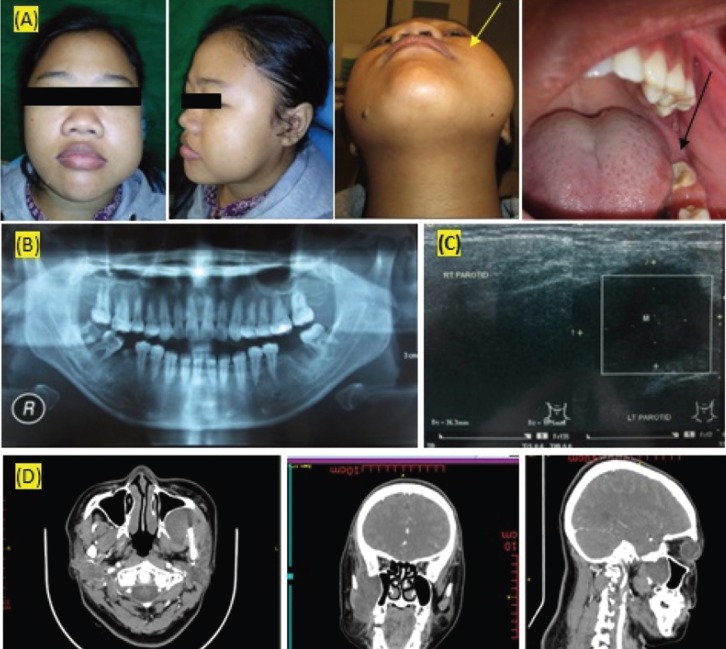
(A) Extra oral picture showed an asymmetry on the left cheek (black arrow) and (B) Orthopantomogram revealed no bone destruction of maxilla and mandible, (C) USG showed a hypoechoid solid mass on the left parotid area. (D) CT scan with contrast enhancement from coronal, axial, and sagital view revealed a slight enhancing soft tissue tumor with well border.
